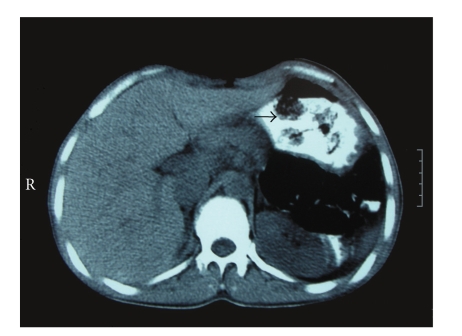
Oral contrast-enhanced CT showing rounded foreign bodies in stomach.
